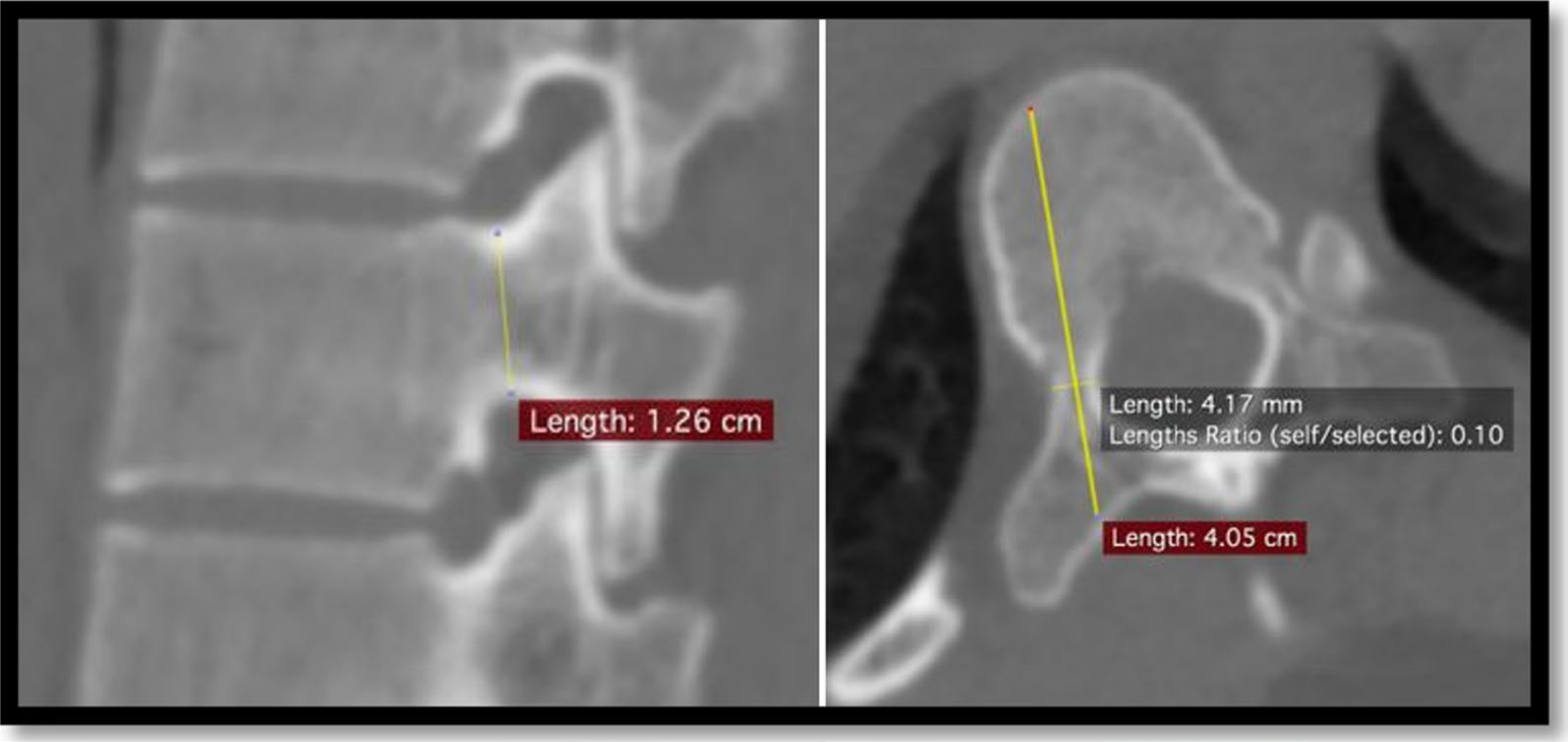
(left) Sagittal CT image depicting pedicle height. (right) Axial CT image depicting pedicle width and depth to anterior cortex. Measurement of 1 vertebrae cranial to apex (T9) in girl with Adolescent Idiopathic Scoliosis Lenke IBN and Cobb angle of 60°
